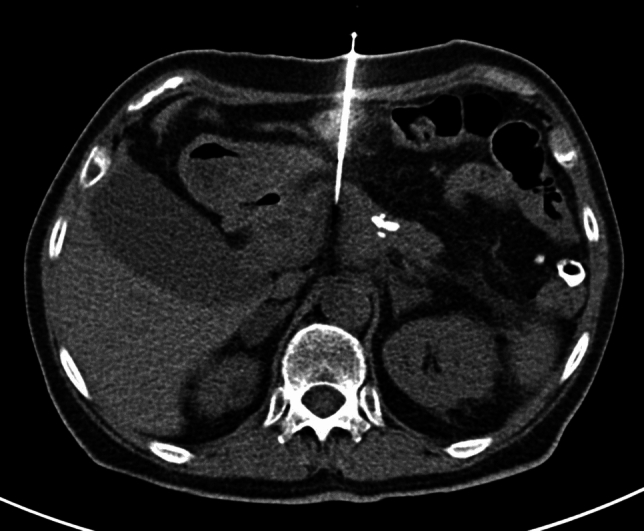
Percutaneous CT-guided biopsy of a pancreatic lesion using a direct anterior access. A non-enhanced axial CT image shows the needle inserted through the anterior abdominal wall, directed toward the lesion in the body of the pancreas
